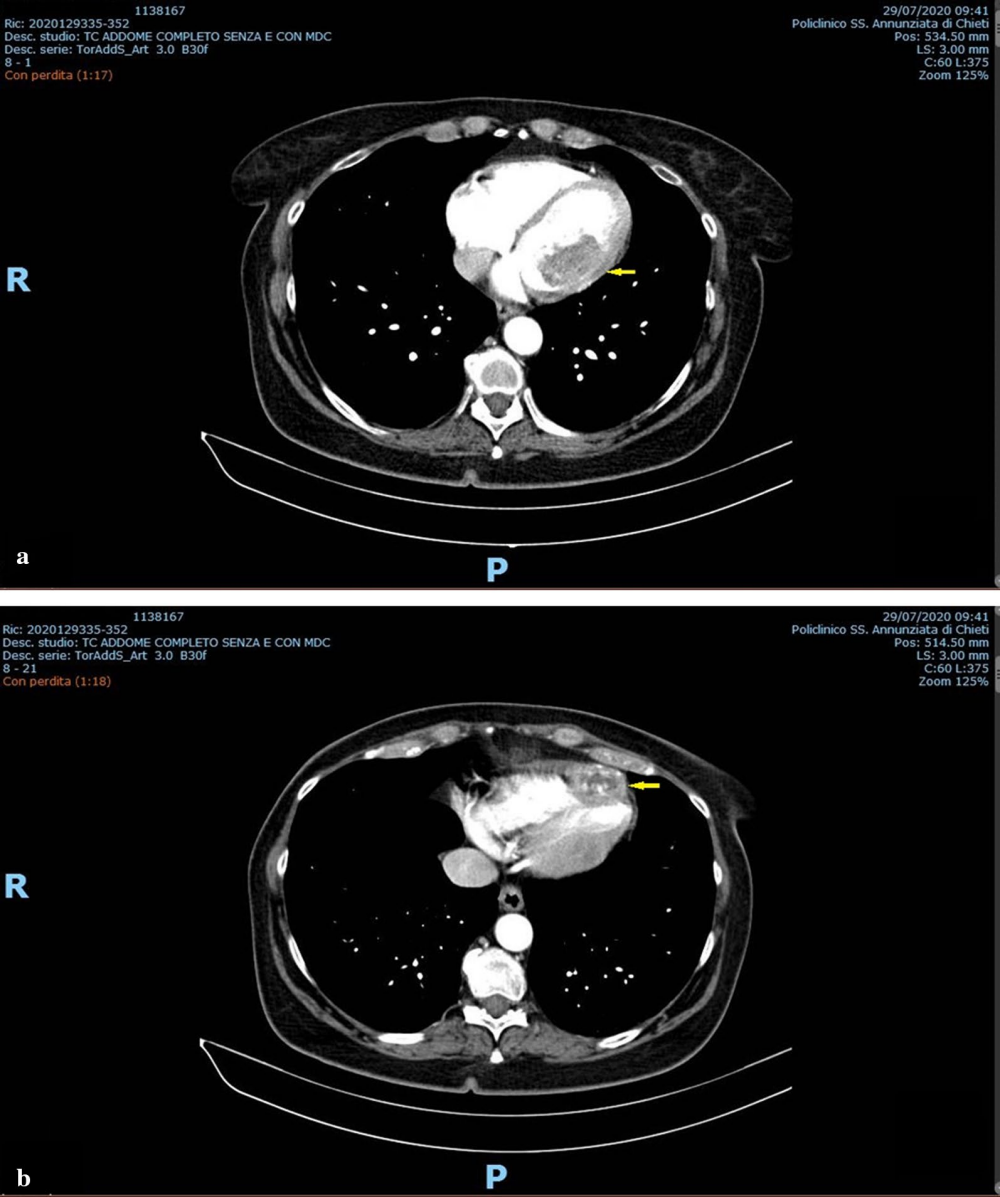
Chest CT scan (July 2020). Intracardiac masses exhibiting inhomogeneous intensity. a Nodule on the lateral wall of the left ventricle (arrow). b Nodule at the apex of the right ventricle (arrow)
